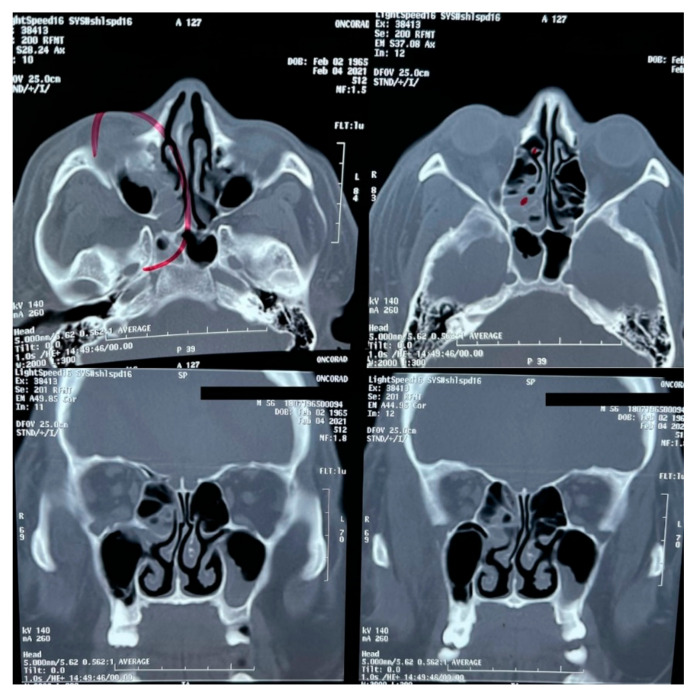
CT-scan of the paranasal sinuses.
